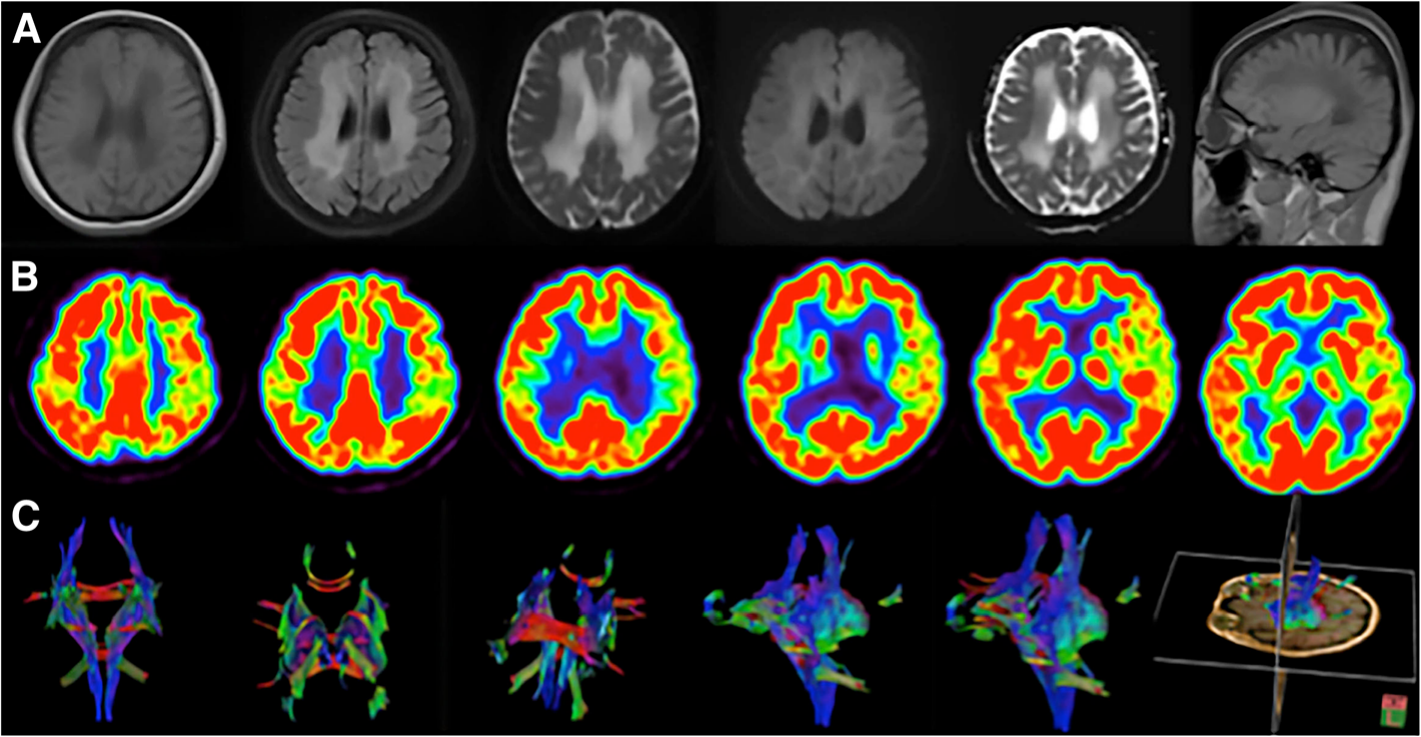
Neuroimaging results of the VWMD patient. a Axial T1-weighted, T2-weighted, Flair, DWI, ADC and coronal T1-weighted images showing extensive cerebral white matter abnormalities with central areas resembling signal intensity of cerebrospinal fluid surrounded by a rim of hyperintensity in the periventricular and subcortical regions. White-matter rarefaction and cystic degeneration are more evident in subcortical and periventricular regions. Obvious brain atrophy was also detected. b Brain ^18^F-FDG PET showed decreased FDG uptake in left frontal, parietal and temporal lobe. Compared with right side, the decline rate is 17, 12 and 17%. c Brain DTI disclosed fibrous white bundle was reduced sparsely among bilateral periventricular
